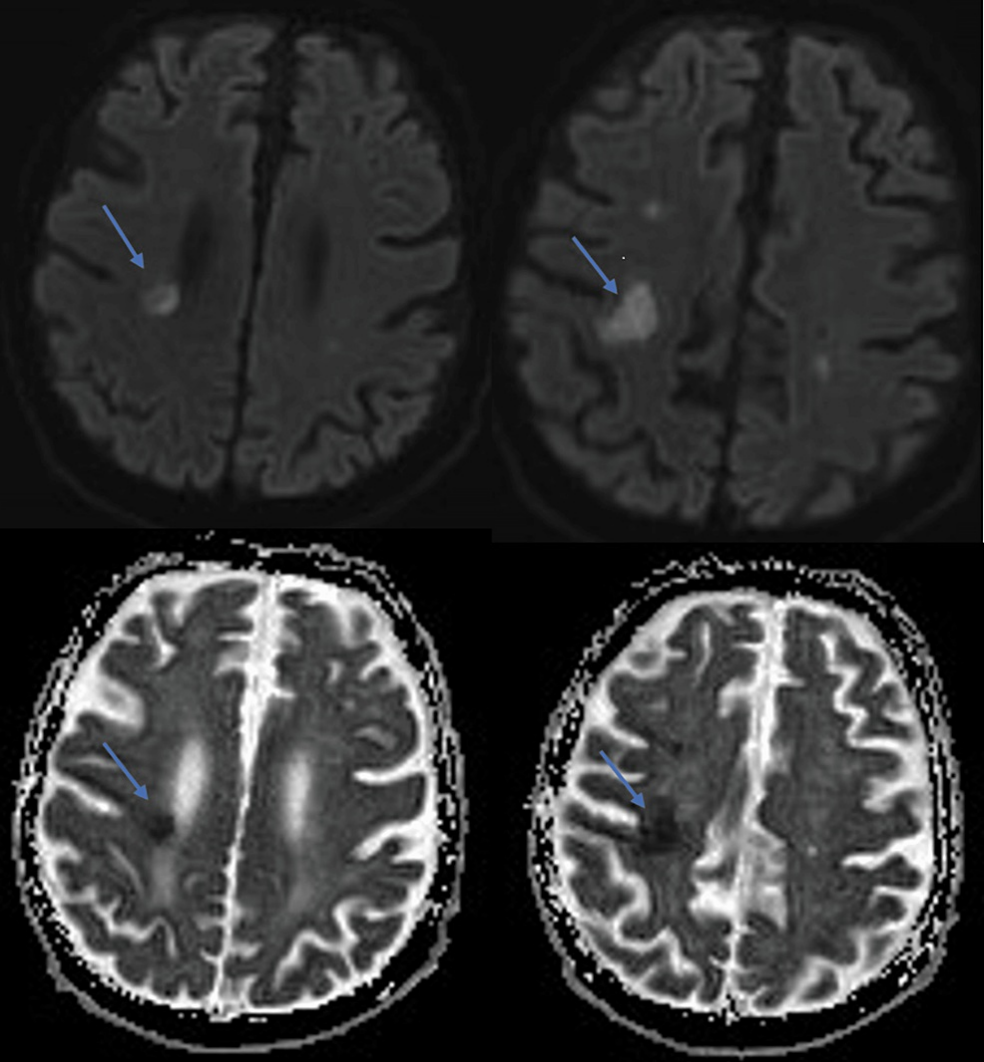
Brain MRI Both axial DWI images (on the top) and ADC map (on the bottom) showed a signal restriction in the right frontoparietal region. DWI: diffusion-weighted imaging; ADC: apparent diffusion coefficient
